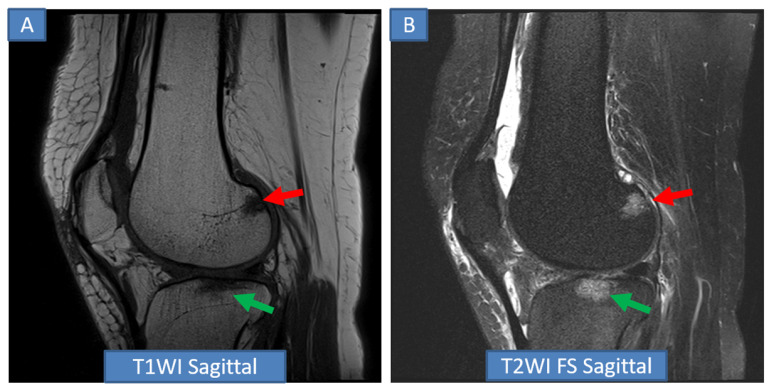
A 46-year-old woman presented with persistent right knee swelling and pain six months after a fall. Initial MRI showed a lateral meniscus tear, which was treated with repair and later meniscectomy with incomplete symptom relief. Physical examination showed swelling extending from the lateral knee to the anterior thigh, with limited extension but no tenderness. Retrospective review of MRI demonstrated edema of the right distal femoral condyle (red arrow) and tibial plateau (green arrow) with osteochondral lesions, which is low signal intensity on the T1W image (A) and high signal intensity on the T2W FS image (B).
